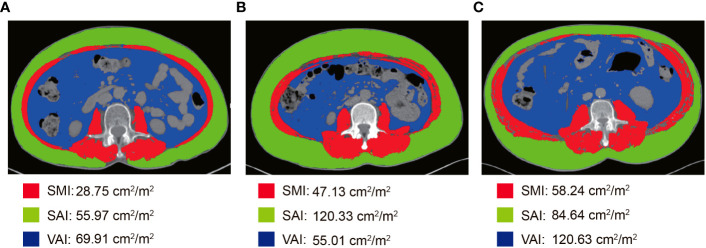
Body composition measurement based on CT images at the level of the third lumbar spine. (A) L-SMI; (B) H-SAI; (C) H-VAI.
